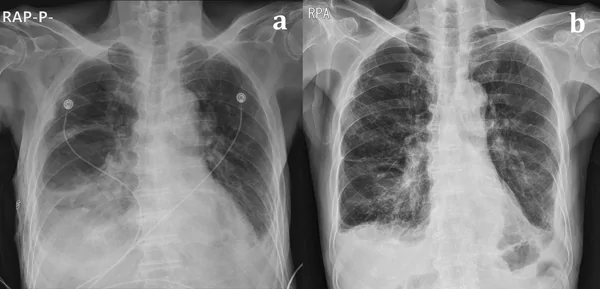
(a) Chest radiograph obtained on postpleurodesis Day 3 showing increased pleural effusion and dense infiltration in the right lower lung field, highly suggestive of acute pneumonitis. (b) Follow‐up chest radiograph showing near‐complete resolution of the effusion and infiltrates after conservative management.
